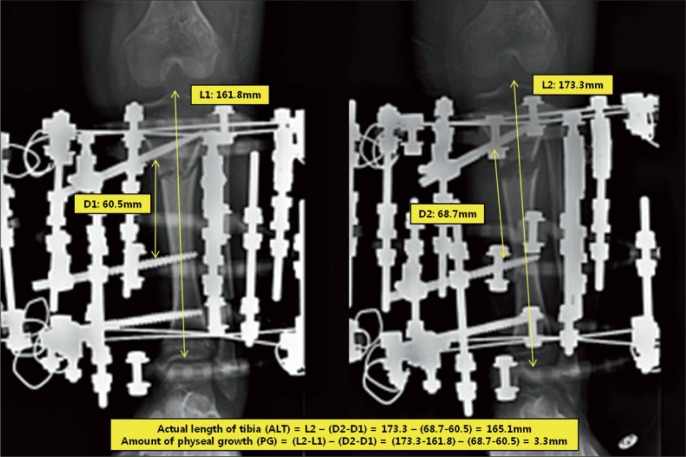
Radiographic measurement of the actual tibial length. Actual length of the tibia is calculated as L2 – (D2 – D1) and the amount of physeal growth is calculated as (L2 – L1) – (D2 – D1) where D1 is the distance between the middle half pins before distraction, D2 is the distance between the middle half pins after distraction, L1 is the length of the tibia before distraction, and L2 is the length of the tibia after distraction.
